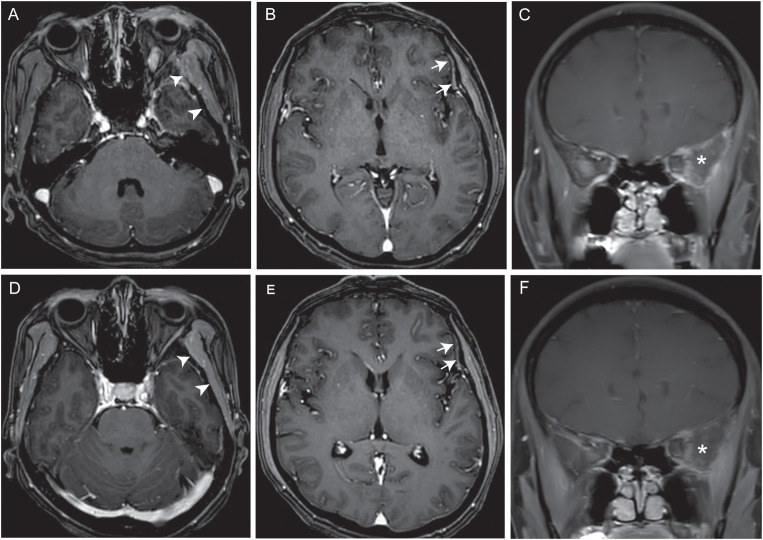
Radiologic response to combination therapy with anlotinib and temozolomide. (A–C) Baseline contrast-enhanced T1-weighted brain MRI before treatment initiation. Axial (A, B) and coronal (C) T1-weighted contrast-enhanced brain MRI images show abnormal enhancement of the left temporalis muscle (A) arrowhead and dura mater (B) arrows, and an enhancing mass centered on the left anterior skull base, intracranial compartment and orbital cavity (C) asterisk. (D–F) Follow-up imaging after 3 months of therapy. Corresponding axial (D, E) and coronal (F) sequences indicate complete resolution of temporalis muscle enhancement (D), arrowhead, normalization of dural signal intensity (E), arrows, and marked reduction in lesional enhancement (F), asterisk.
